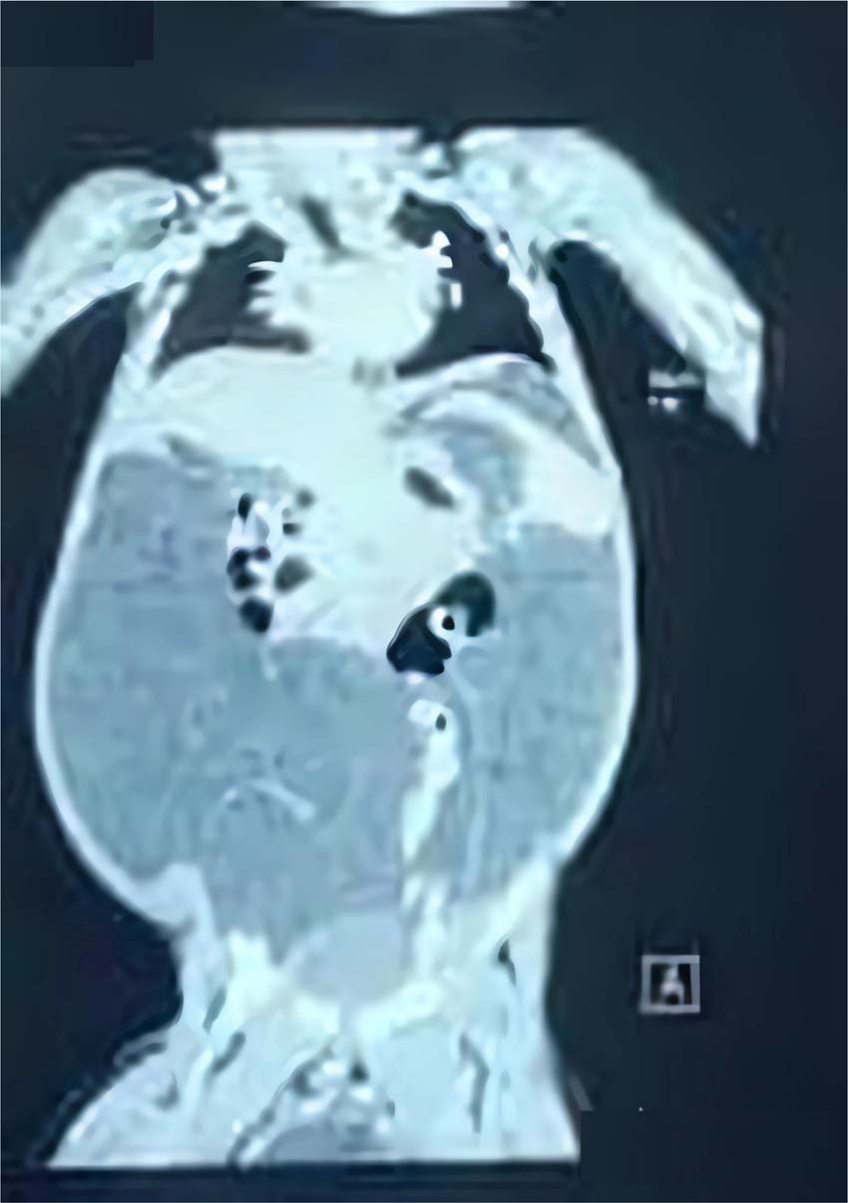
coronal view of CT abdomen and pelvic demonstrates the scrotal extent of abdominopelvic cystic lesion
